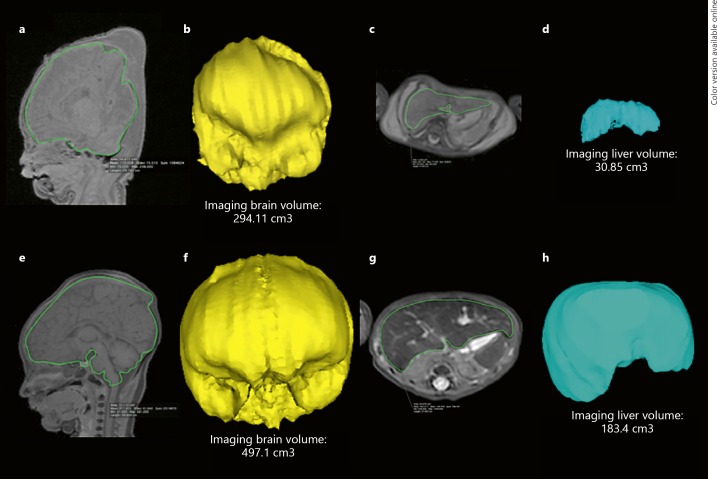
PMMR of a stillborn 39-week gestational age fetus with FGR (a–d) and a stillborn 40-week non-FGR (control) patient (e–h). MRI manual segmentation on isovolumetric T1-weighted sequences of the brain are shown in sagittal views (a, e, green outline) with corresponding derived 3D volumes (b, f). A similar method was adopted on isovolumetric T2-weighted imaging of the abdomen for the liver in axial sections (c, g, green outline) with corresponding derived 3D volumes (d, h). For the fetus with FGR (a–d), the IB: LvR was calculated as 9.53 (AB: LwR was 7.6), and for the control fetus without FGR (e–h) the IB: LvR was 2.7 (AB: LwR was 2.4).
